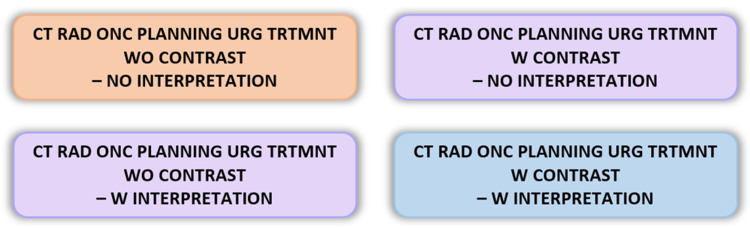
Optimized CT order names based on presence or absence of IV contrast and formal radiology interpretation. RAD ONC: radiation oncology; URG TRTMNT: urgent treatment; W: with; WO: without
